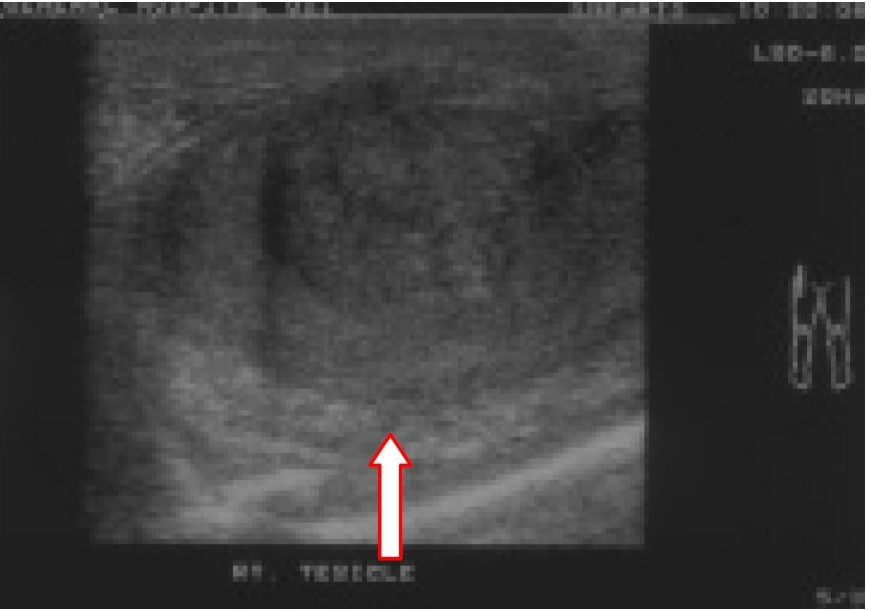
Ultrasound right testis demonstrating loss of tissue plane between testis and adjacent structures/overlying skin (arrow).
